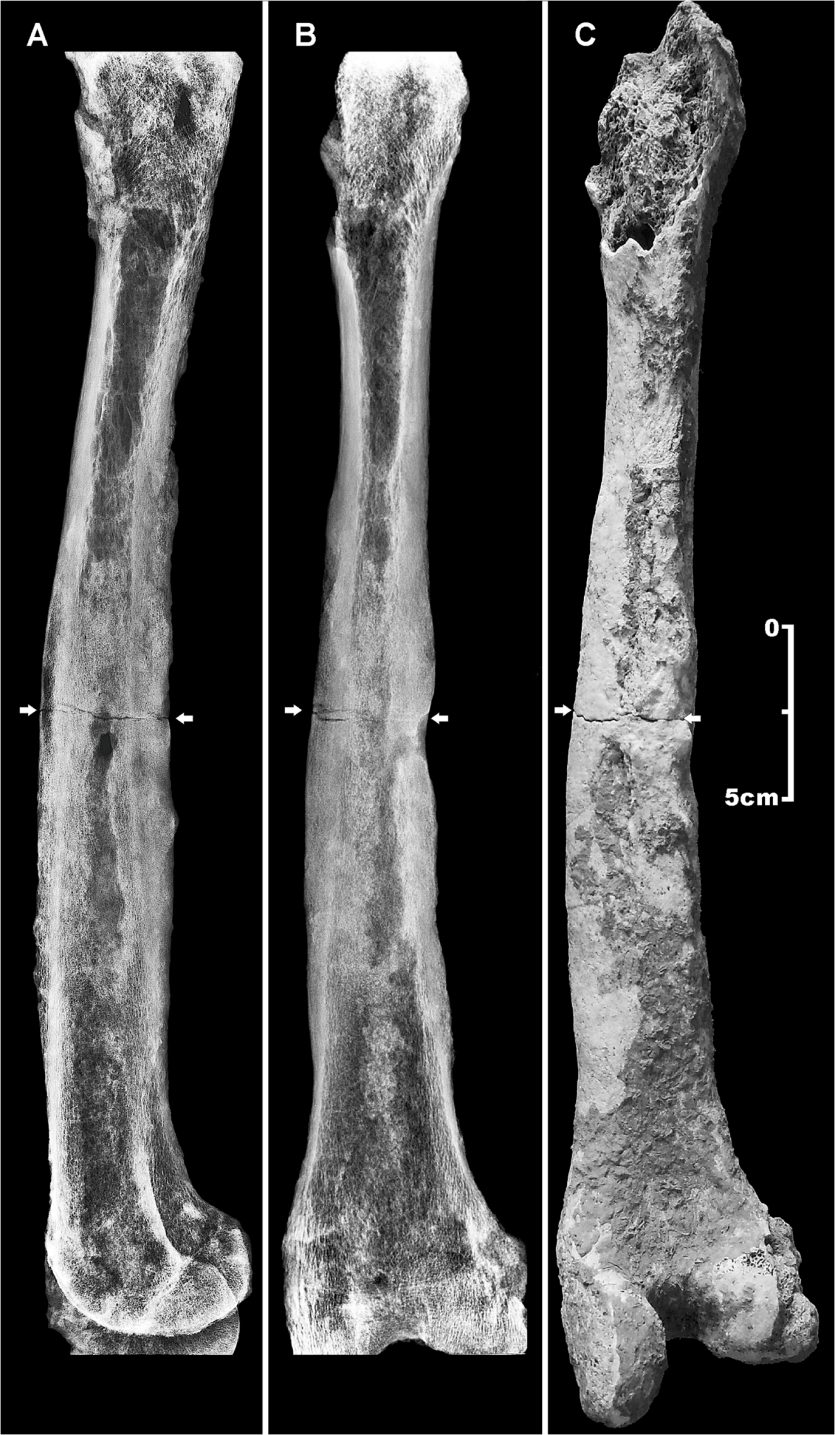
Traumatic and osteomyelitic femoral lesions. Latero-lateral (A) and antero-posterior (B) X-ray images, and posterior view (C) of the right femur (A, B). Diffuse periosteal new bone apposition (*involucrum*) testified by a more radiolucent enlarged cortical bone layer, a reduced medullary cavity, and a cloaca drainage canal, diagnostic of long-term chronic osteomyelitis (C). Abnormal size of the femoral shaft induced by periosteal new bone apposition. At the mid-shaft, the fracture line and the cloaca opening are evident (arrows). Scale bar equals 5 cm.
